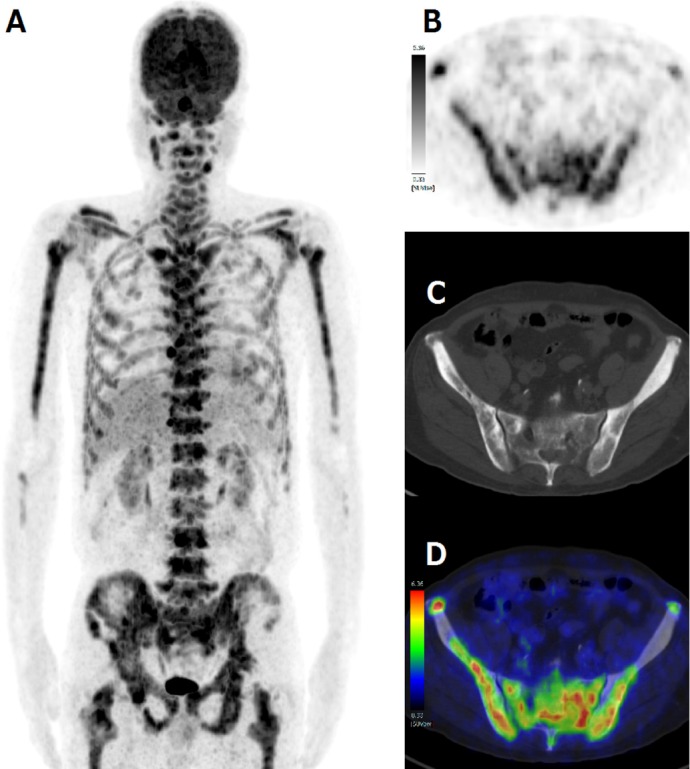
F-18 FDG PET maximum intensity projection (MIP) image (A), axial pelvic PET (B), CT (C), and fused PET/CT (D) at 4 weeks after the third injection of Ra-223 therapy. Diffuse FDG uptake in the bone without osteoblastic lesions shows active osteolytic metastases or bone marrow involvement. Osteoblastic lesions without FDG correspond to lesions with decreased HMDP uptake.
